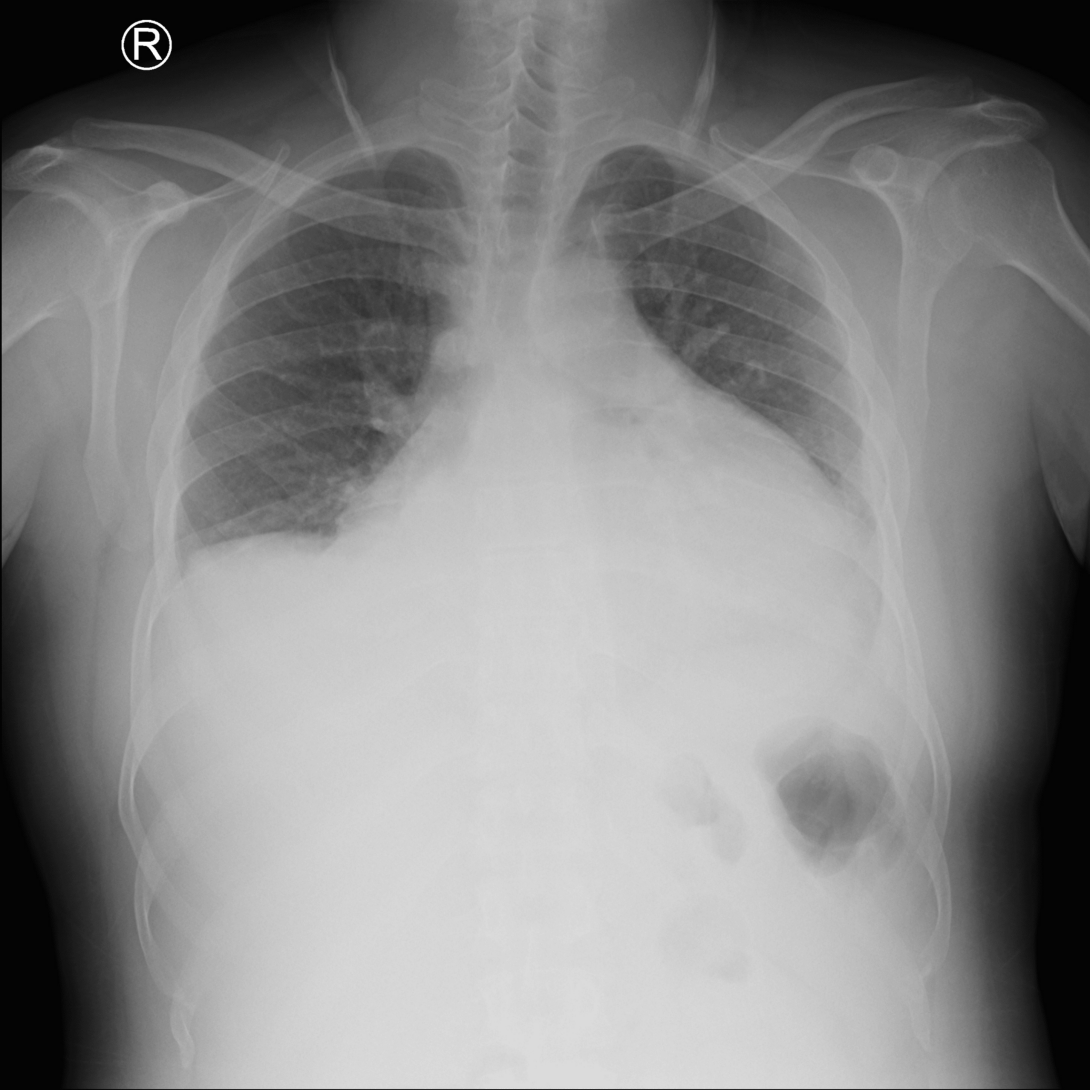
Chest X-ray showing cardiomegaly
